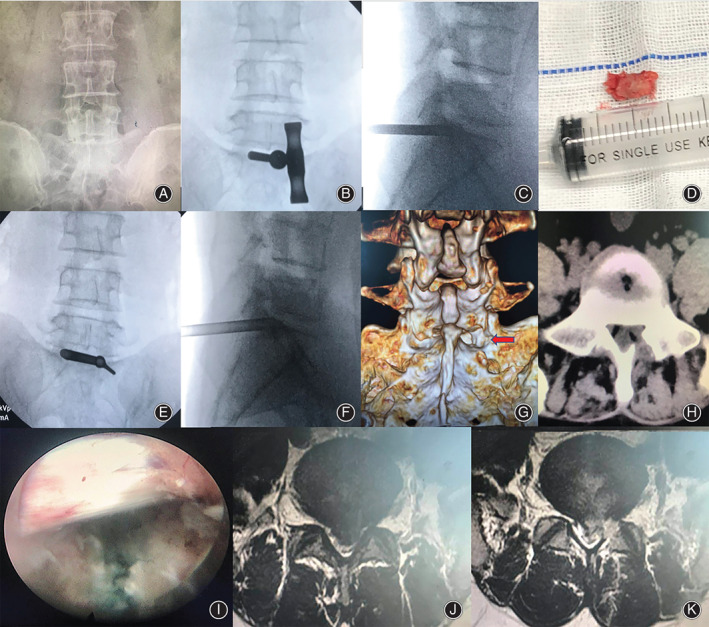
Typical case one. (A) Lumbar anterior–posterior X‐ray image. Narrow interlaminar window of L_5_S_1_ level. (B and C) The trephine was used for laminoplasty at the safety zone. (D) Resected bone from the safety zone. (E and F) Working cannula was inserted at the safety zone. (G and H) Postoperative CT scan revealed laminoplasty (arrow). (I) S_1_ nerve root was completely released. (J and K) Preoperative and postoperative MRI showed removal and good decompression of the S_1_ nerve root and dura.
